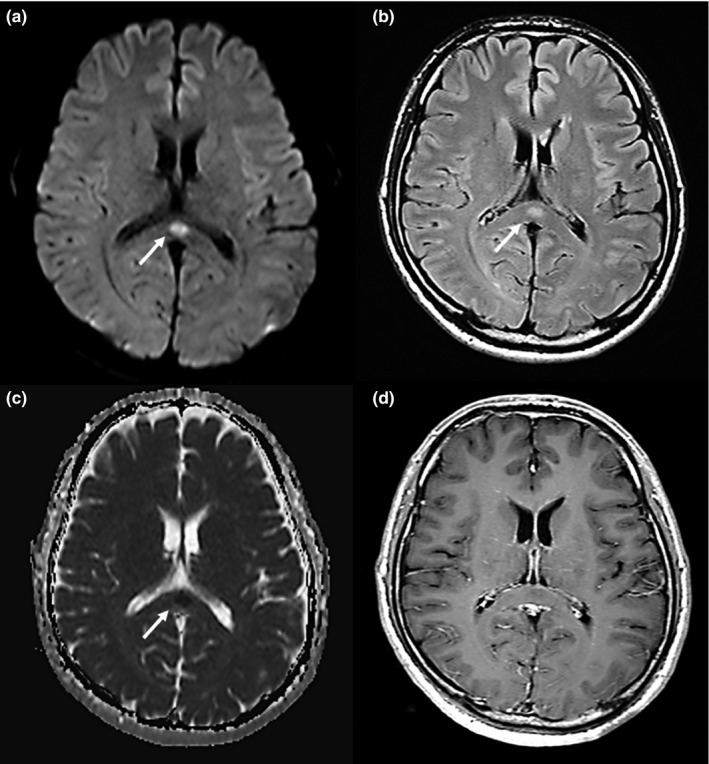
A 41‐year‐old man was admitted with fever, headache, and fatigue. He received a diagnosis of clinically mild encephalitis. Magnetic resonance imaging was performed on day 1 hospitalization. Diffusion‐weighted (a) and fluid‐attenuated inversion recovery (b) imaging showed a marked hyperintense signal (arrows) on the splenium of the corpus callosum (SCC). The apparent diffusion coefficient (c) showed a hypointense signal (arrow). T1‐weighted imaging with contrast material (d) showed an isointense signal that was not enhanced by the contrast material. Arrows indicate the SCC
